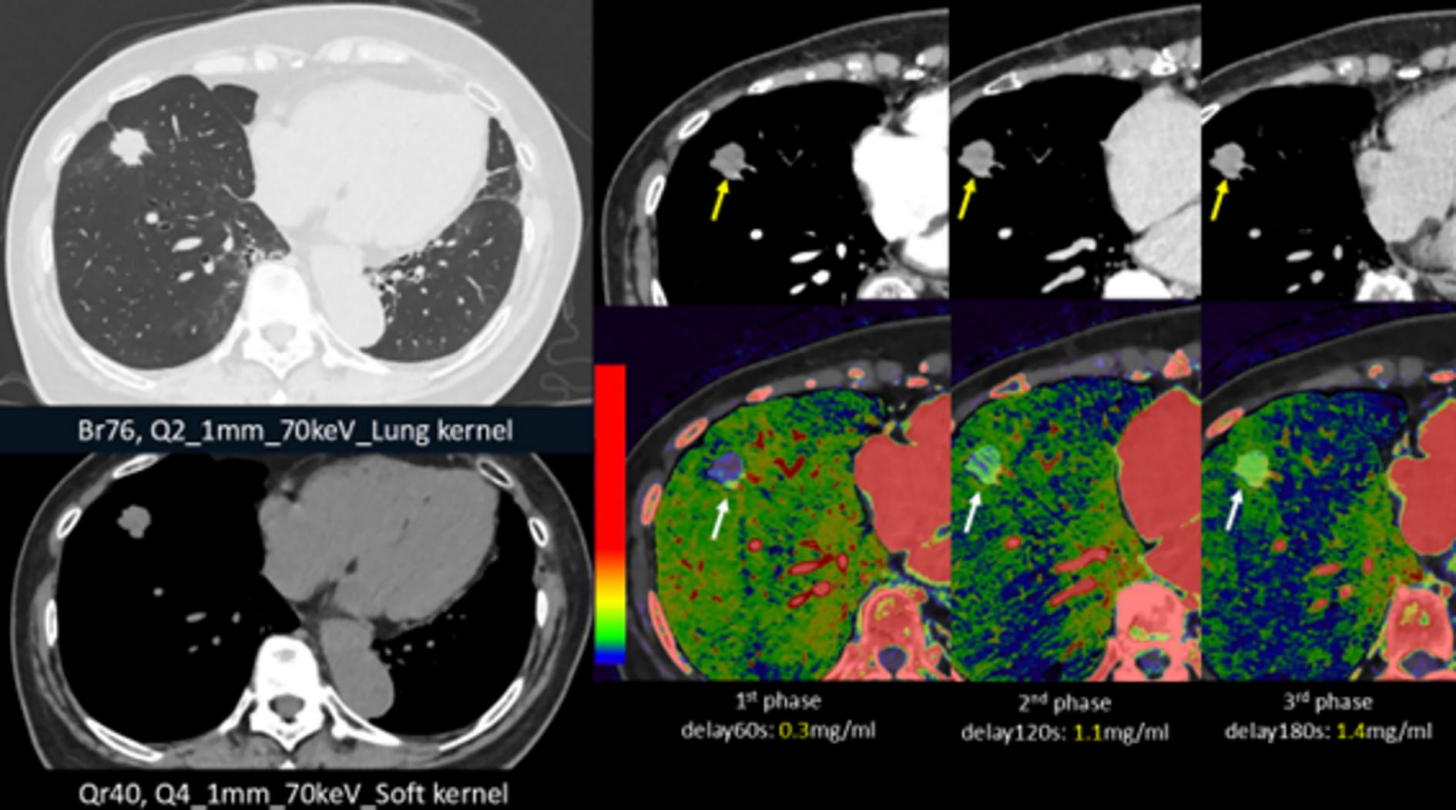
PC-CT can provide high spatial resolution and precise iodine mapping at the same time. Utilizing PC-CT in oncology has the potential to enhance lung cancer staging, therapy planning, and treatment response assessment, as well as detecting incidental pulmonary nodules by improving subjective and objective evaluations
